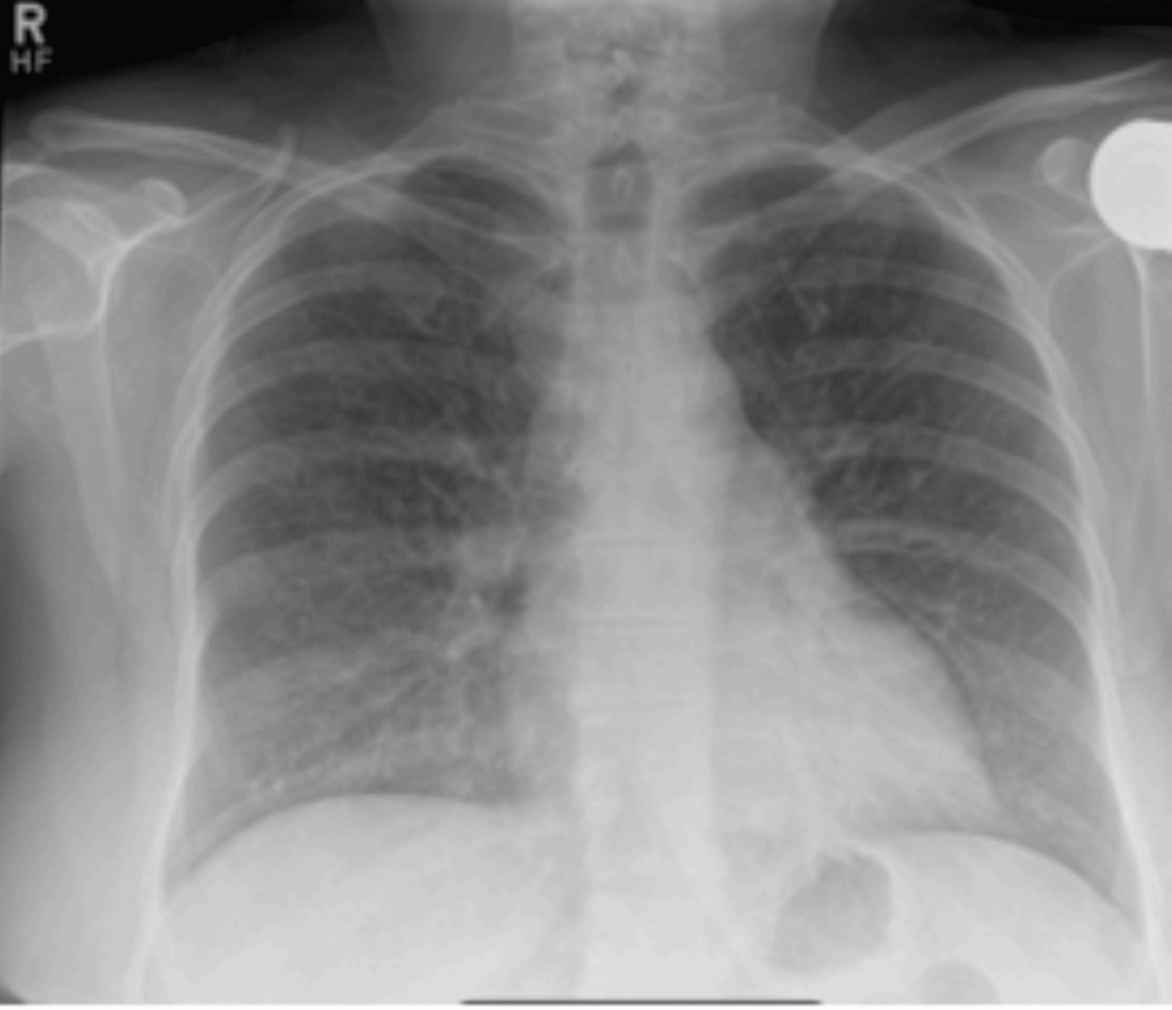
Bilateral infiltrates noted on the chest X-ray
